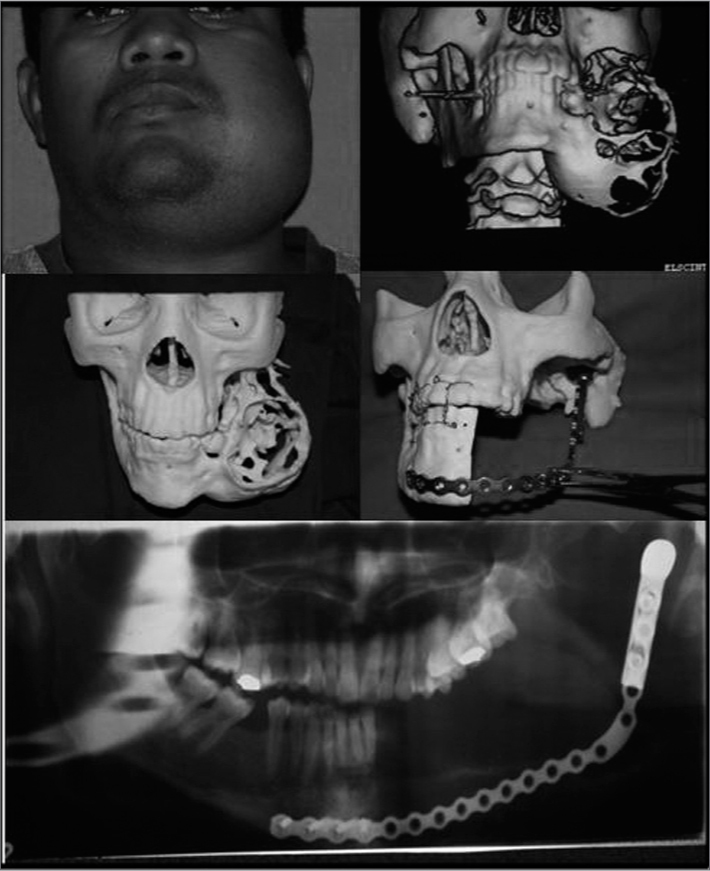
Postoperative clinical and radiographic aspects, prototyping model and postoperative radiographic aspect.
